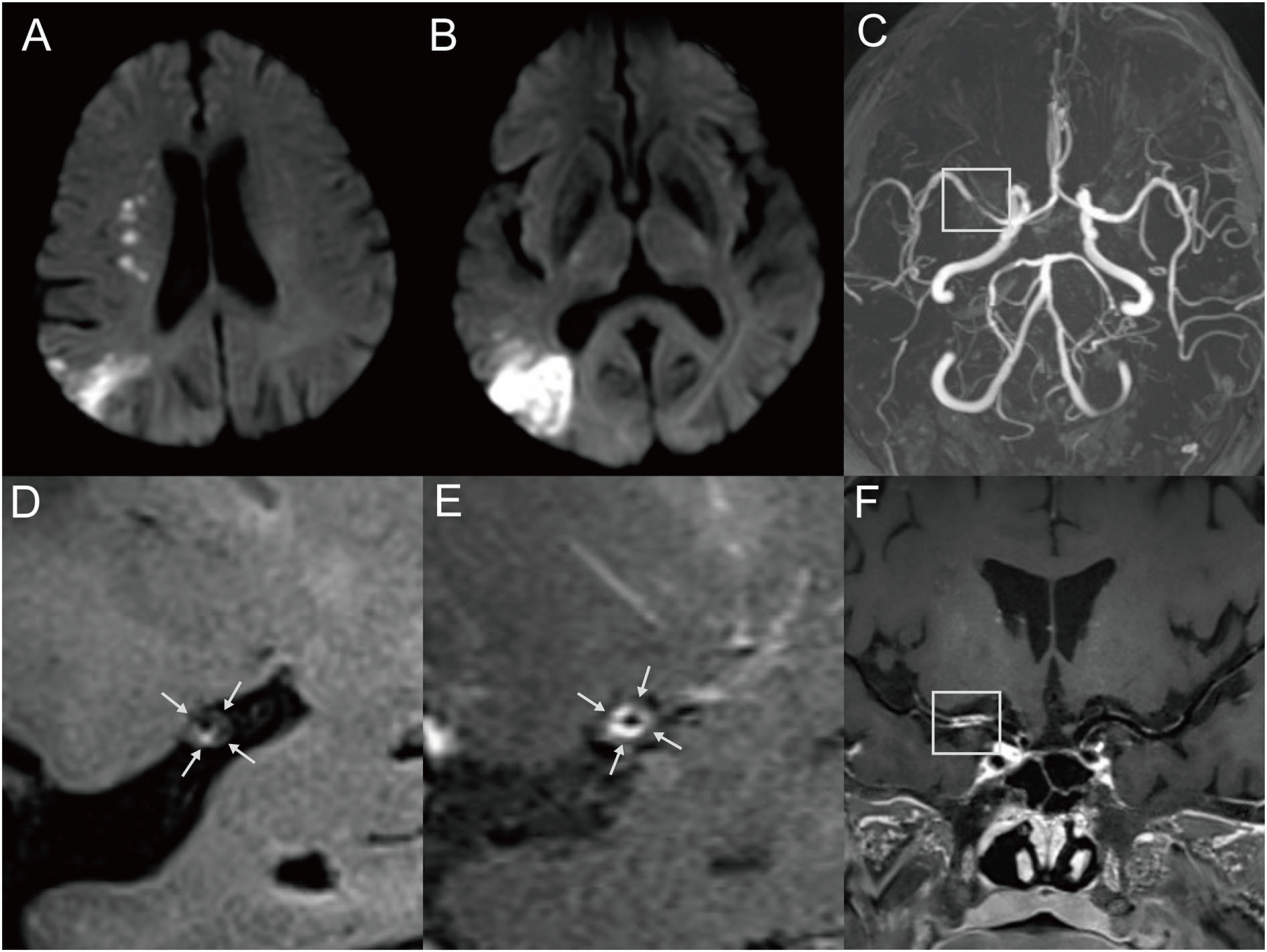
Representative MR images of a 60-year-old male patient with multiple MCA infarctions. (A,B) DWI showed chain-like internal border-zone infarctions and wedge-shaped or spotty cortical infarctions in the right MCA territory. The patient with a cigarette smoking history presented with left-side weakness and mouth askew for 4 days. (C) 3D-TOF-MRA demonstrated severe stenosis (box) of the right MCA. (D) Pre-contrast HRMRI showed a diffuse distributive plaque with a spotted high signal (intraplaque hemorrhage) at the inferior wall of MCA (arrow). (E) Postcontrast HRMRI demonstrated obvious plaque enhancement (arrow). (F) The postcontrast T1-weighted image by curved planar reformation showed a diffuse plaque located at the proximal M1 segment of the right MCA (box).
